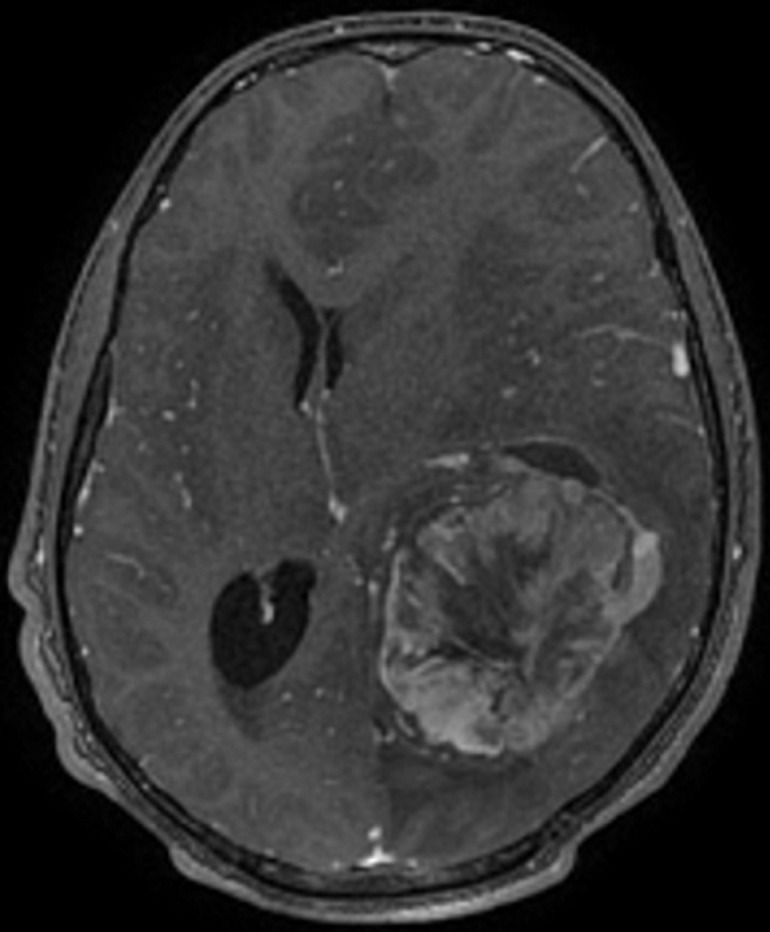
Magnetic resonance imaging (MRI) T1, objectified a left temporo-parieto-occipital intraaxial
